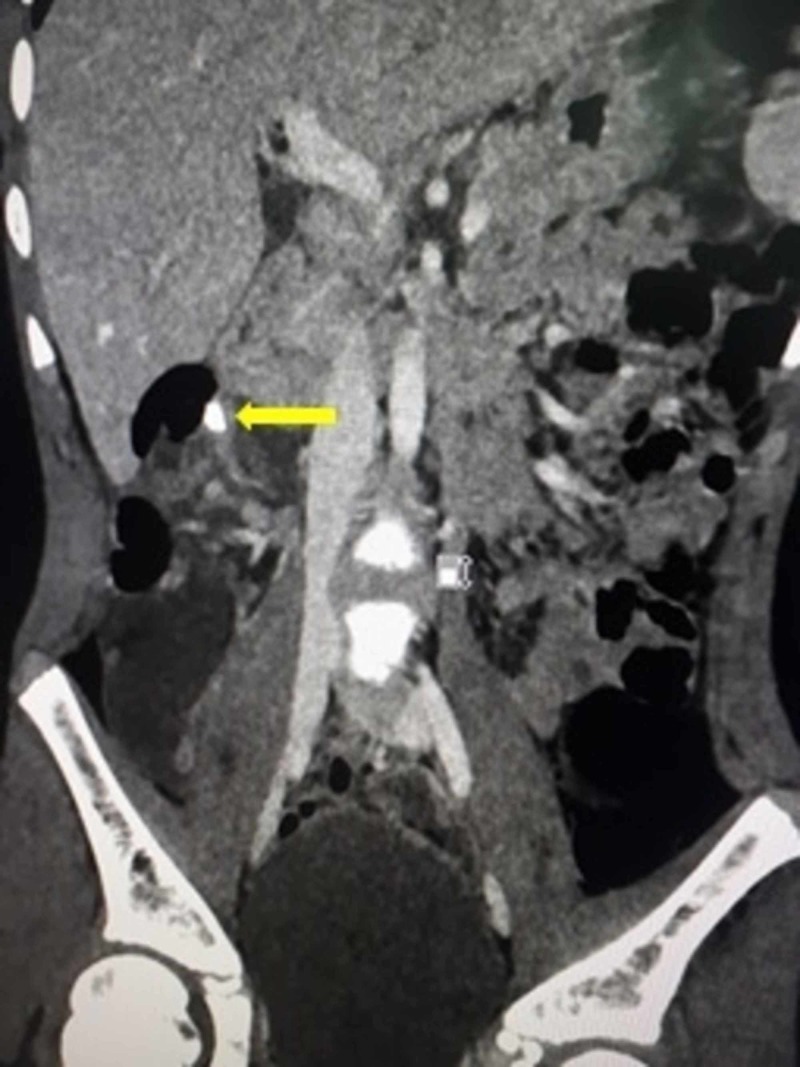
Coronoal CT scan of the abdomen depicting a subhepatic appendicolith (yellow arrow).
